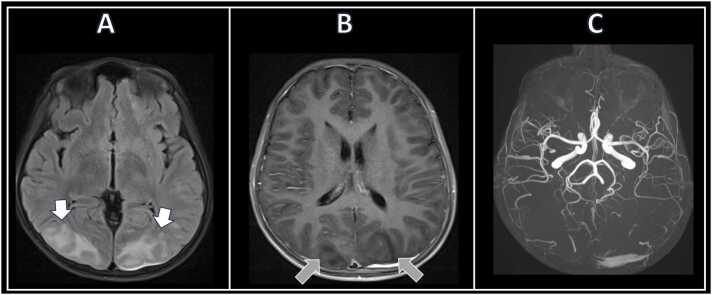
Magnetic resonance imaging of the patient when he developed posterior reversible encephalopathy syndrome. Note the white matter hyperintensities (white arrow) in the FLAIR (subset A) sequence and the corresponding hypo-intensities (grey arrow) in the T1 (subset B) sequence. The subset C depicts the magnetic resonance angiogram. There were no changes of cerebral vasculitis. Only the occipital lobe involvement is shown. FLAIR: Fluid-attenuated inversion recovery.
